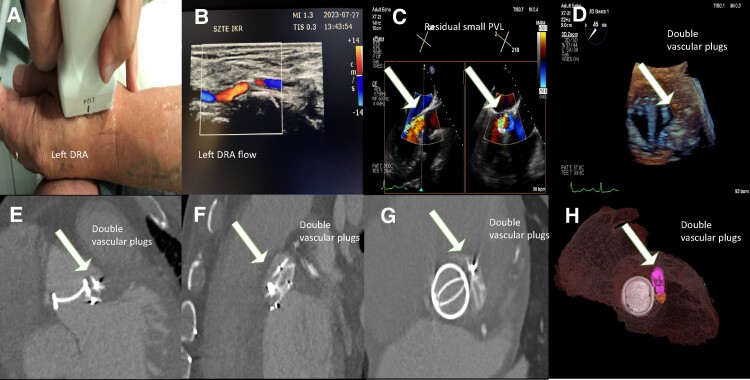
(*A*) An image demonstrating an ultrasound check of the distal radial artery 9 months after leak closure in the anatomical snuffbox. (*B*) A 9-month follow-up of the patency of the access site (the left distal radial artery) for the paravalvular leak closure procedure. (*C*) A transoesophageal echocardiography image at the 9-month follow-up after transcatheter paravalvular leak closure. The white arrows indicate a haemodynamically non-significant residual leak. (*D*) A three-dimensional transoesophageal echocardiography image at the 9-month follow-up after transcatheter paravalvular leak closure. Good mechanical valve leaflet function. A white arrow shows the treated leak position. (*E*, *F*) Computed tomography images demonstrating patent double plug positions in the prior leak location. The white arrows point at the implanted AVPIII plugs. (*G*) A computed tomography image demonstrates a patent plug position (white arrow). Good mechanical valve function. (*H*) A three-dimensional colour reconstruction of the implanted AVPIII plugs.
